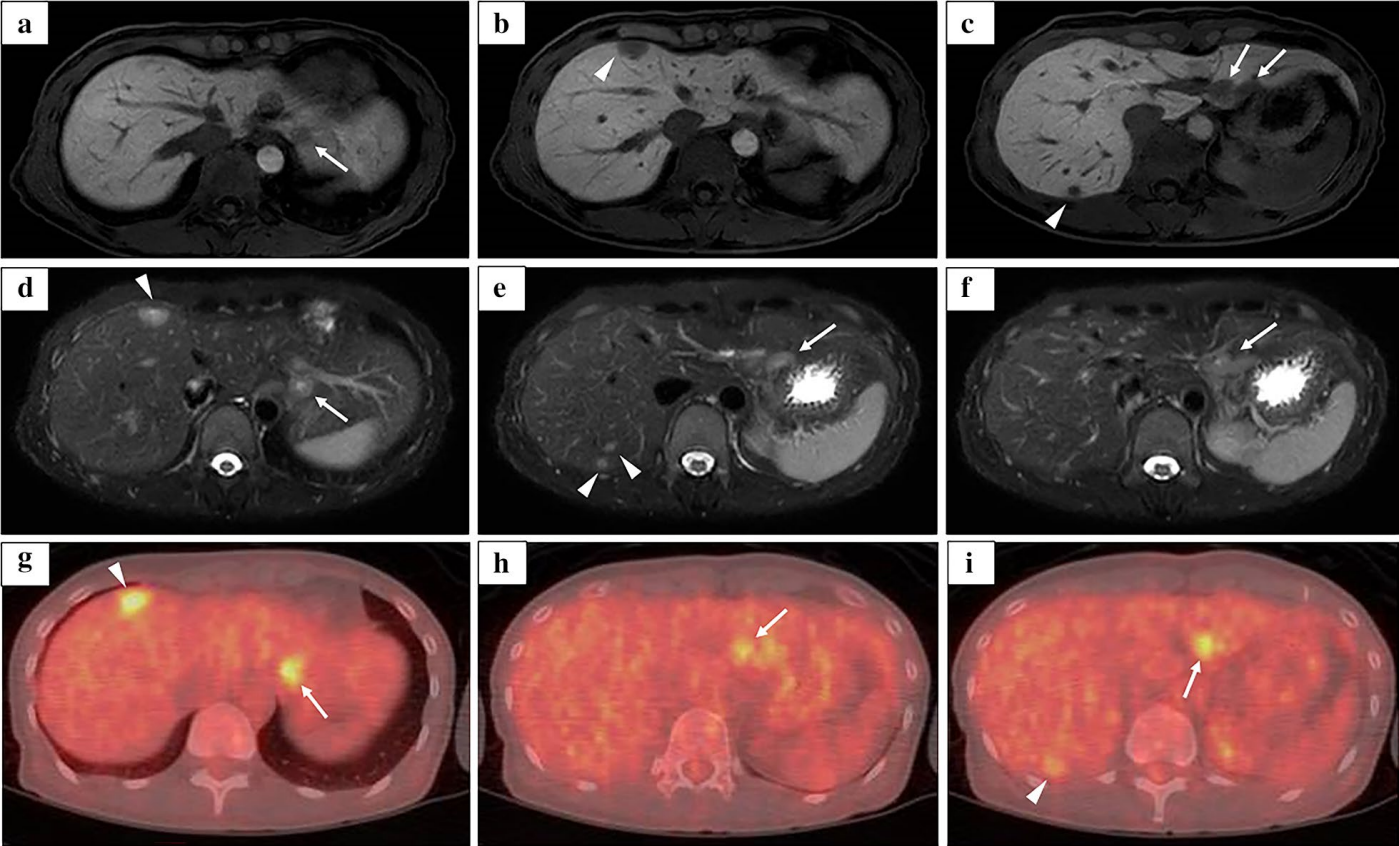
a–c Gadolinium–ethoxybenzyl–diethylenetriamine–pentaacetic acid (Gd–EOB–DTPA) enhanced magnetic resonance imaging (MRI) revealed five tumors including a 15-mm tumor in the lateral superior segment (S2) (a, arrow), a 20-mm tumor in the anterior superior segment (S8) (b, arrowhead), a 16-mm (c, arrow) tumor and a 20-mm tumor (c, arrow) in the lateral inferior segment (S3), a 10-mm tumor in the posterior superior segment (S7) (c, arrowhead). d–f T2-weighted MRI revealed six tumors including a 15-mm tumor in S2 (d, arrow), a 16-mm (e, arrow) tumor and a 20-mm tumor (f, arrow) in S3, a 3-mm tumor and a 10-mm tumor in S7 (e, arrowheads), and a 20-mm tumor in S8 (d, arrowhead). A typical target sign was observed in the tumors in S2 (d, arrow) and S8 (d, arrowhead). g–i Enhanced 2-[^18^F] fluoro-2-deoxy-d-glucose (FDG) positron emission tomography showed FDG uptake in all tumors except one 3-mm tumor in S7
